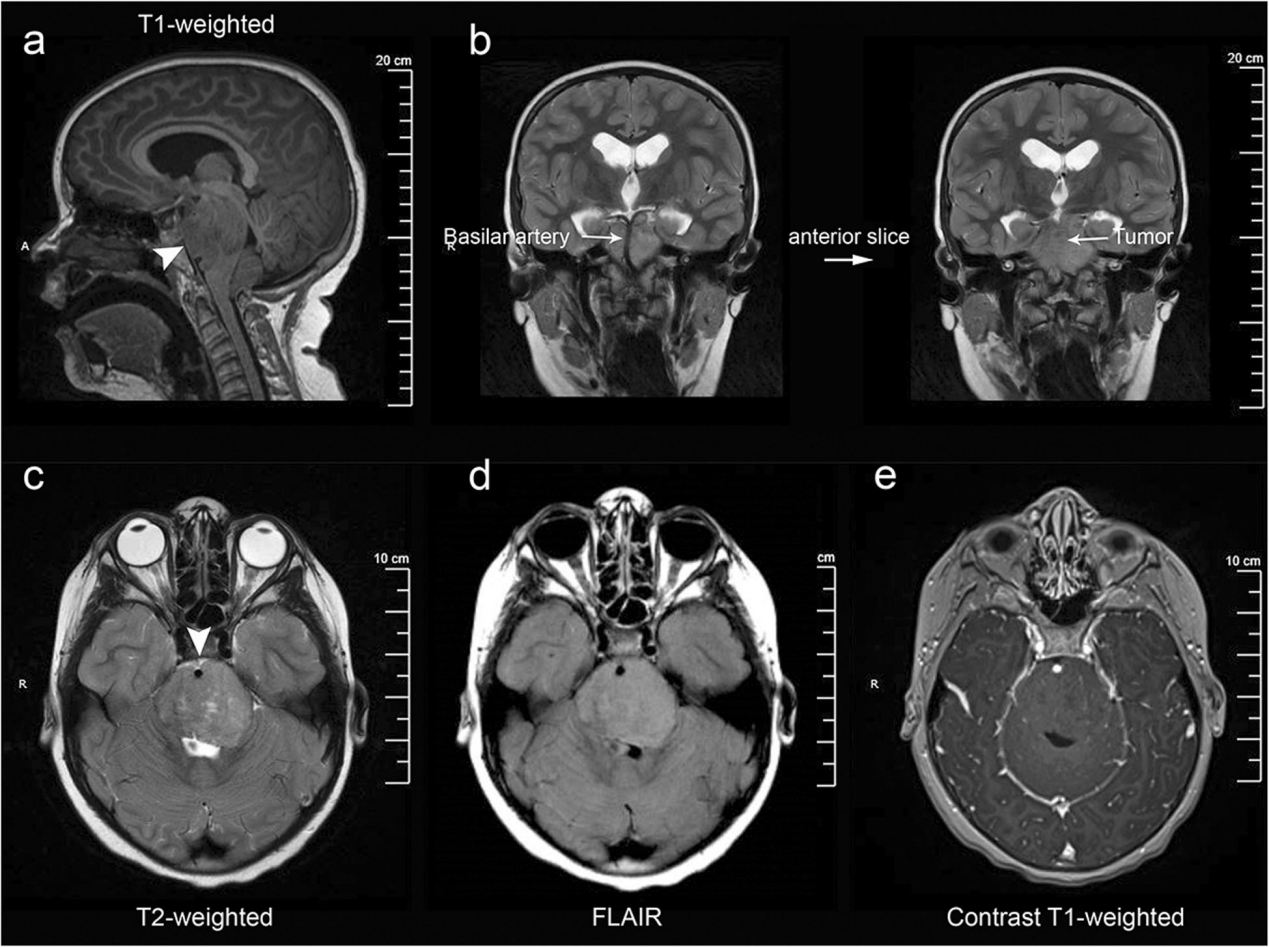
Representative MRI characteristics. T1-weighted sagittal image showed an infiltrative mass centered within and expanding the pons (a). The exophytic portion of the tumor is seen encasing the basilar artery (b). The mass demonstrated classic MR characteristics of DIPG including increased T2 (c) and FLAIR (d) signals, and lack of post contrast enhancement (e)
